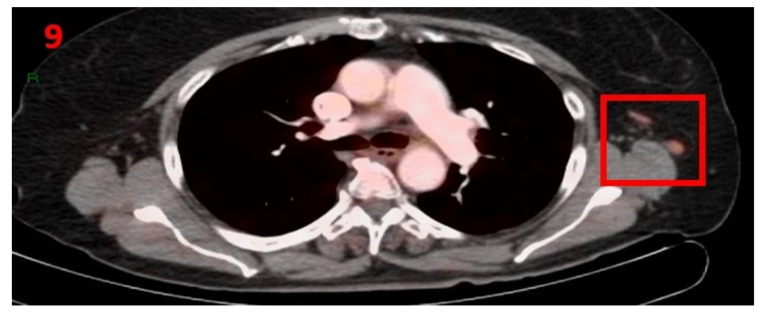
[18F]FDG PET-CT showing hypermetabolic uptake in the 9th patient with lymphadenopathy in the left axillary region (red square).
